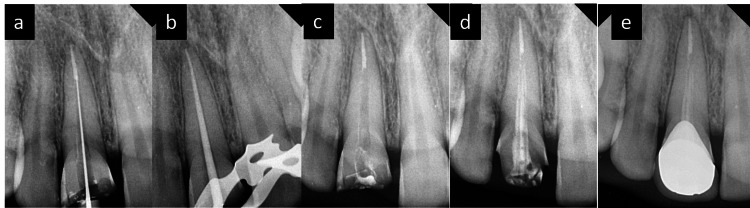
(a) Intraoral periapical (IOPA) radiograph showing the working length determination of tooth 11. (b) IOPA radiograph showing the master cone fit in tooth 11. (c) IOPA radiograph showing sectional obturation in tooth 11. (d) IOPA radiograph of tooth 11 showing the placement of Dentapreg PINPosts assembly inside the canal and cemented using dual-cure resin cement. (e) IOPA radiograph of tooth 11 post-crown cementation.
